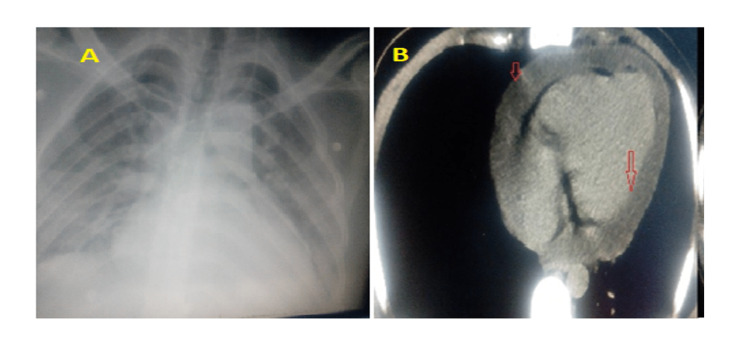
(A) CXR shows cardiomegaly and clear lungs. (B) CT chest showing massive circumferential pericardial effusion (red arrows).
